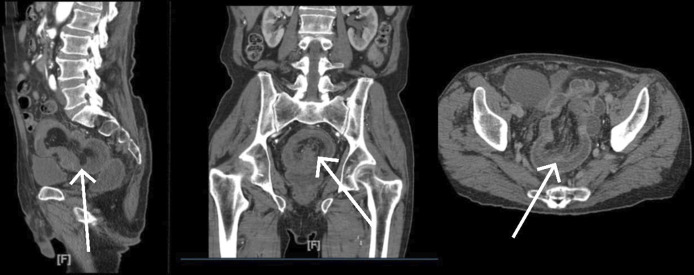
Multiple computed tomography scan views showing a distended rectosigmoid and rectum with target sign at the rectosigmoid junction consistent with intussusception.
